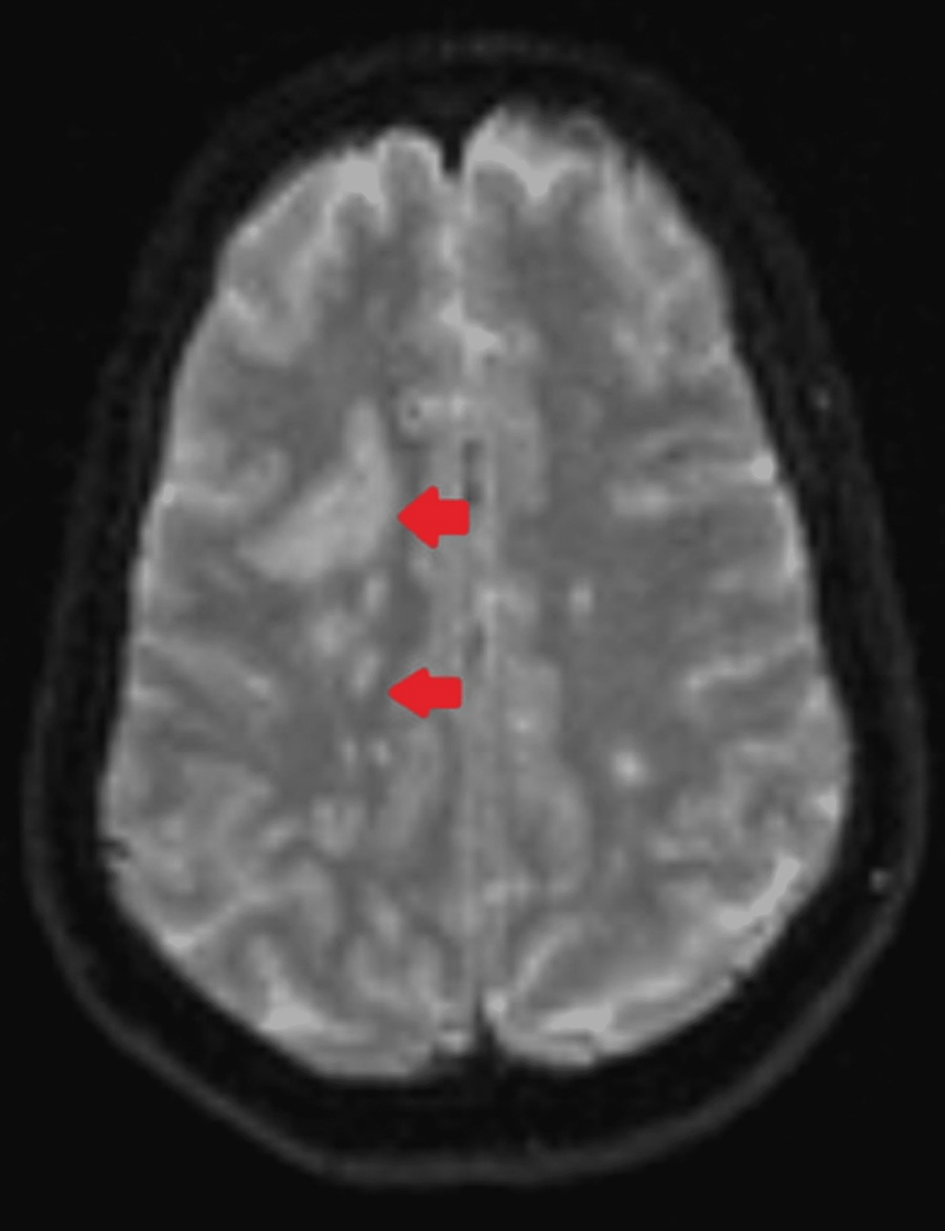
Diffusion-weighted MRI showing diffusion restriction in the lesions (red arrows).
